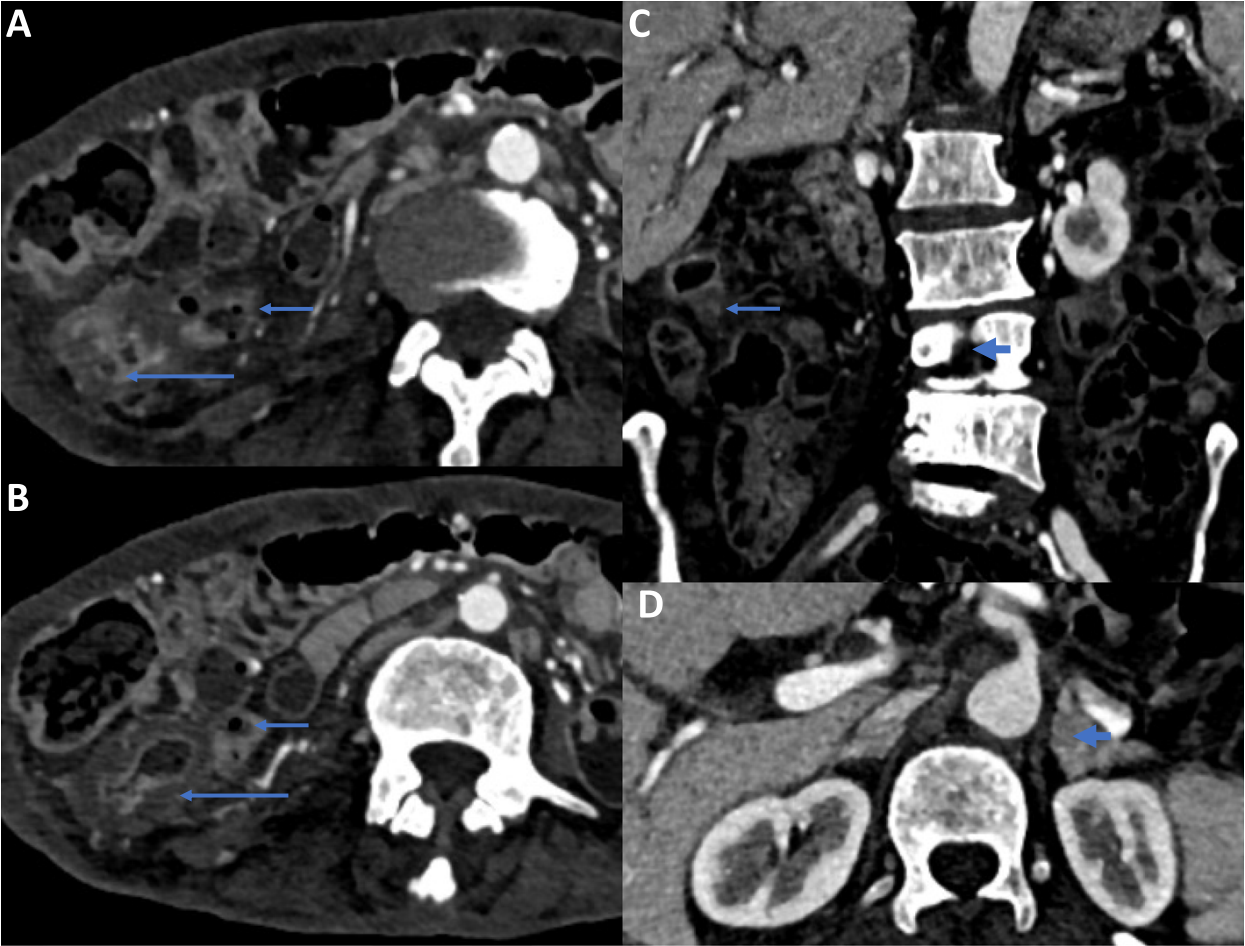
Axial (A, B) and coronal (C) contrast enhanced CT enterography images show contiguous asymmetric circumferential mural thickening of the caecum (arrows, A and B) and terminal ileum (short arrows, A-C). The caecum is pulled up in subhepatic location (not shown). Also note the lumbar vertebral lesion (arrow head). Axial CT image at the level of adrenals shows bulky and heterogeneous left adrenal gland (arrow head) suggestive of granulomatous involvement. The right adrenal gland was also involved (not shown). The patient was diagnosed to have disseminated tuberculosis
